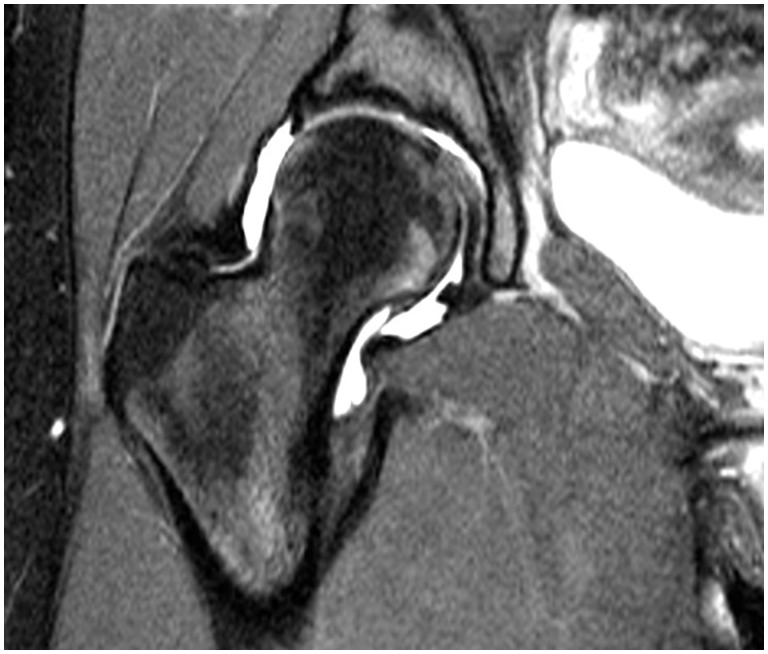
Appearance of an intact LT on MRI.
